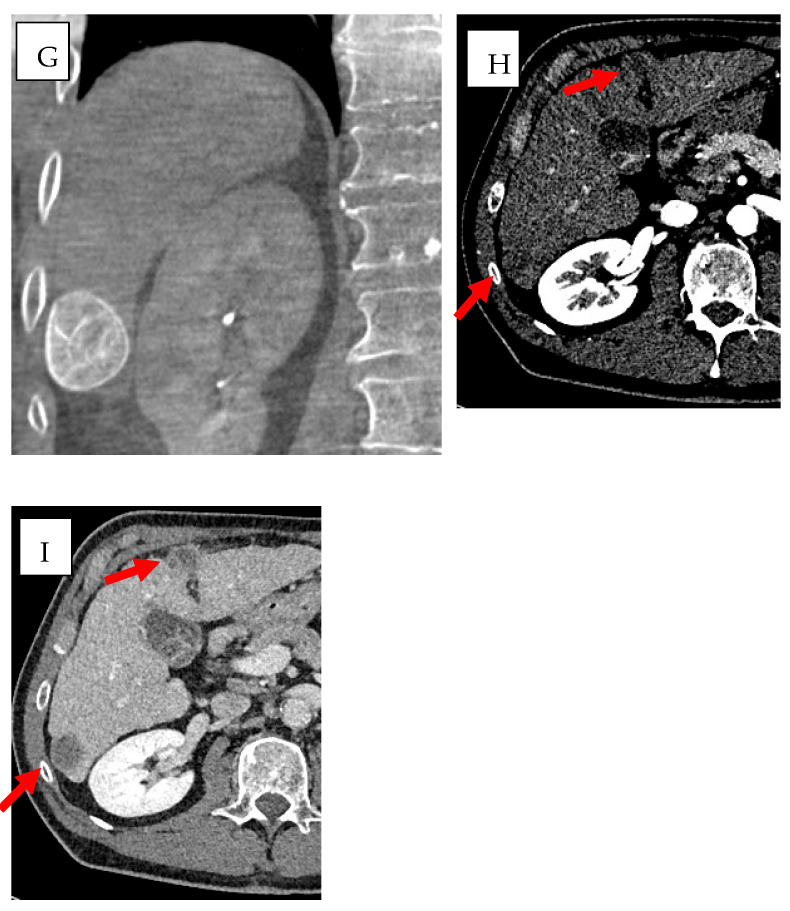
Multifocal HCC in a patient with HBV/HDV/alcohol-related cirrhosis (BCLC stage B) treated with DEB-TACE using BioPearl (Tokyo, Japan). (A,B) Pre-treatment CT shows two subcapsular lesions (~3 cm) in segments VI and IV with (A) arterial hyperenhancement and (B) venous washout (red circles), consistent with HCC. (C,D) Emboguide (Version 1.2.1, Philips) software and CBCT mapping of the arterial pathway from the right hepatic artery to the target lesion in segment IV. (E) Angiographic image showing the lesion in segment IV supplied by the left hepatic artery. (F,G) Emboguide (Philips) software and CBCT mapping of the right hepatic artery pathway to the lesion in segment VI before chemoembolization, showing enhancement of the target area. (H,I) Post-treatment CT in (H) arterial and (I) venous phases demonstrates hypodense areas (~3.5 cm) in segments VI and IV, consistent with treated lesions (red arrows).
